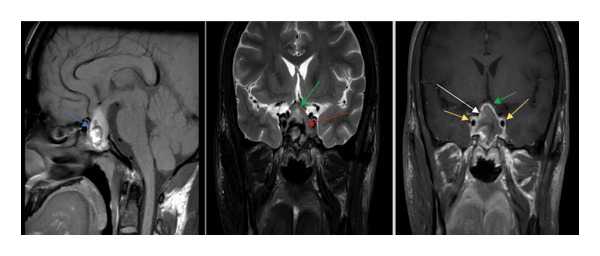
MRI pituitary with contrast (preoperative). Selective images from pituitary MRI scan (sagittal T1WI, coronal T2WI, and postgadolinium coronal image) show a large heterogeneous sellar and suprasellar lesion. It appears heterogeneously hyperintense on T1WI (blue arrow), corresponding to iso‐ to slightly hyperintense on T2WI, with internal focal dark or hypointense signal (red arrow), and shows mild enhancement with more obvious peripheral enhancement (white arrow). The lesion depresses the sphenoid sinus, laterally displaces the carotid arteries bilaterally (yellow arrow), and extends superiorly, elevating the optic chiasm (green arrow).
